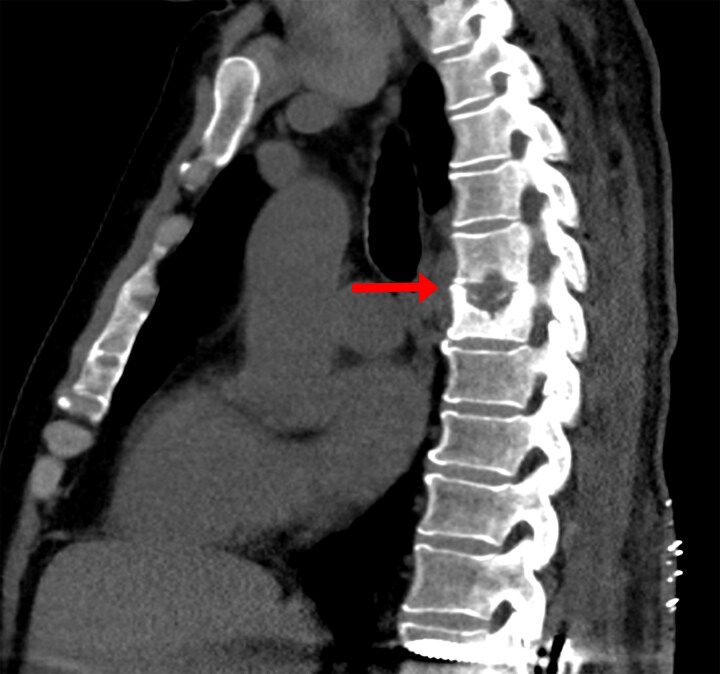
Noncontrast chest computed tomography in a sagittal view. The arrow highlights incidentally discovered lytic destruction of the T6 and T7 vertebral bodies.
